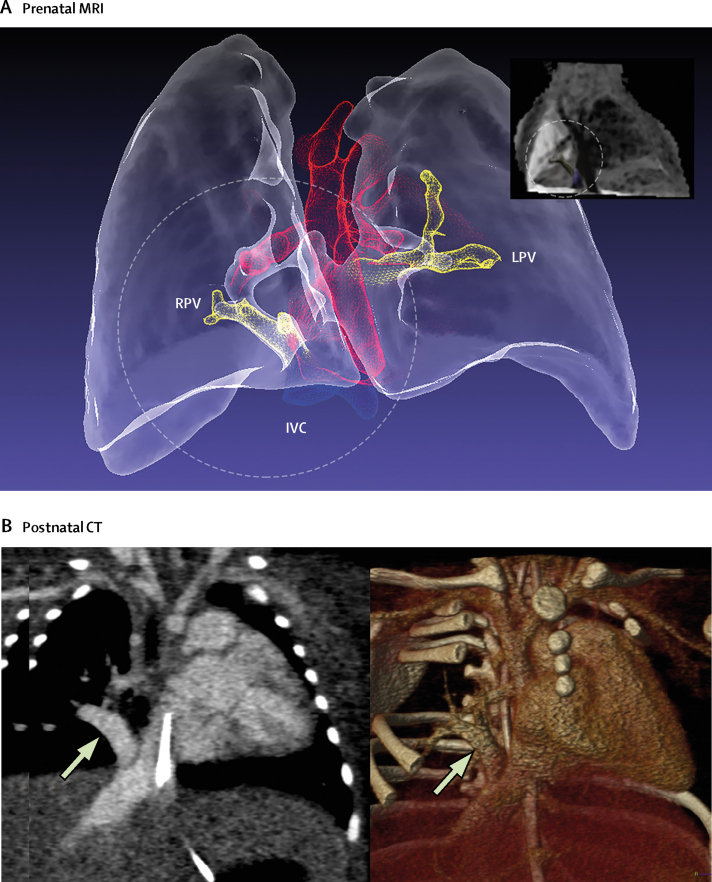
Example segmentation from motion-corrected 3D data, in a 33-week fetus referred because of abnormal right pulmonary veins on echocardiography (A) In this anterior projection of the lungs and major blood vessels, a single right pulmonary vein (RPV) can be seen draining anomalously to the inferior vena cava (IVC) in the circled area. A single left pulmonary vein (LPV) was also noted. Inset: minimum-intensity projection of the 0·7mm isotropic volume used to generate segmentation (RPV=yellow, IVC=blue). (B) All findings were confirmed postnatally by contrast-enhanced CT, with the anomalous vein indicated with an arrow. See video 4 for more detail.
